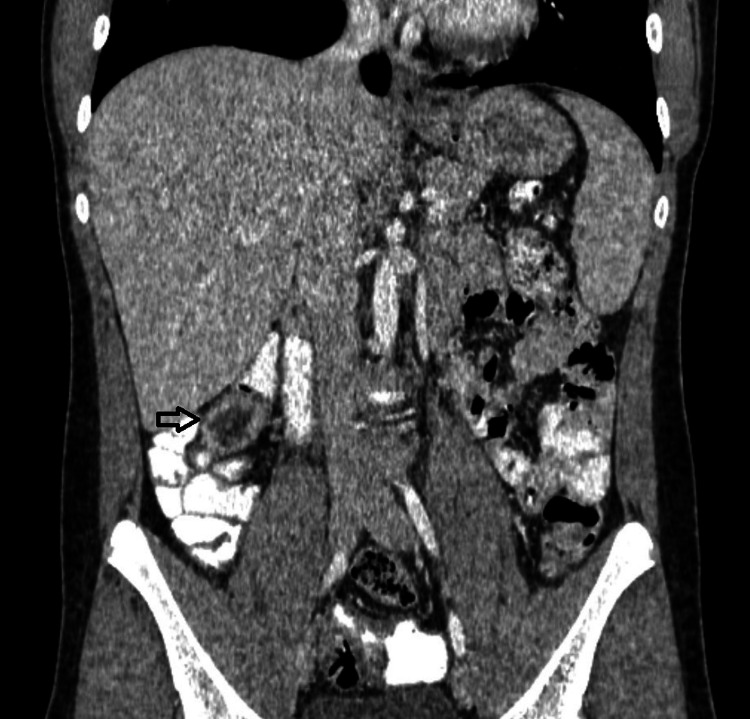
Enhanced CT with oral contrast, coronary view showing a tubular formation located beneath the liver, enhancing through the arterial phase, suggesting an inflamed Meckel’s diverticulum
